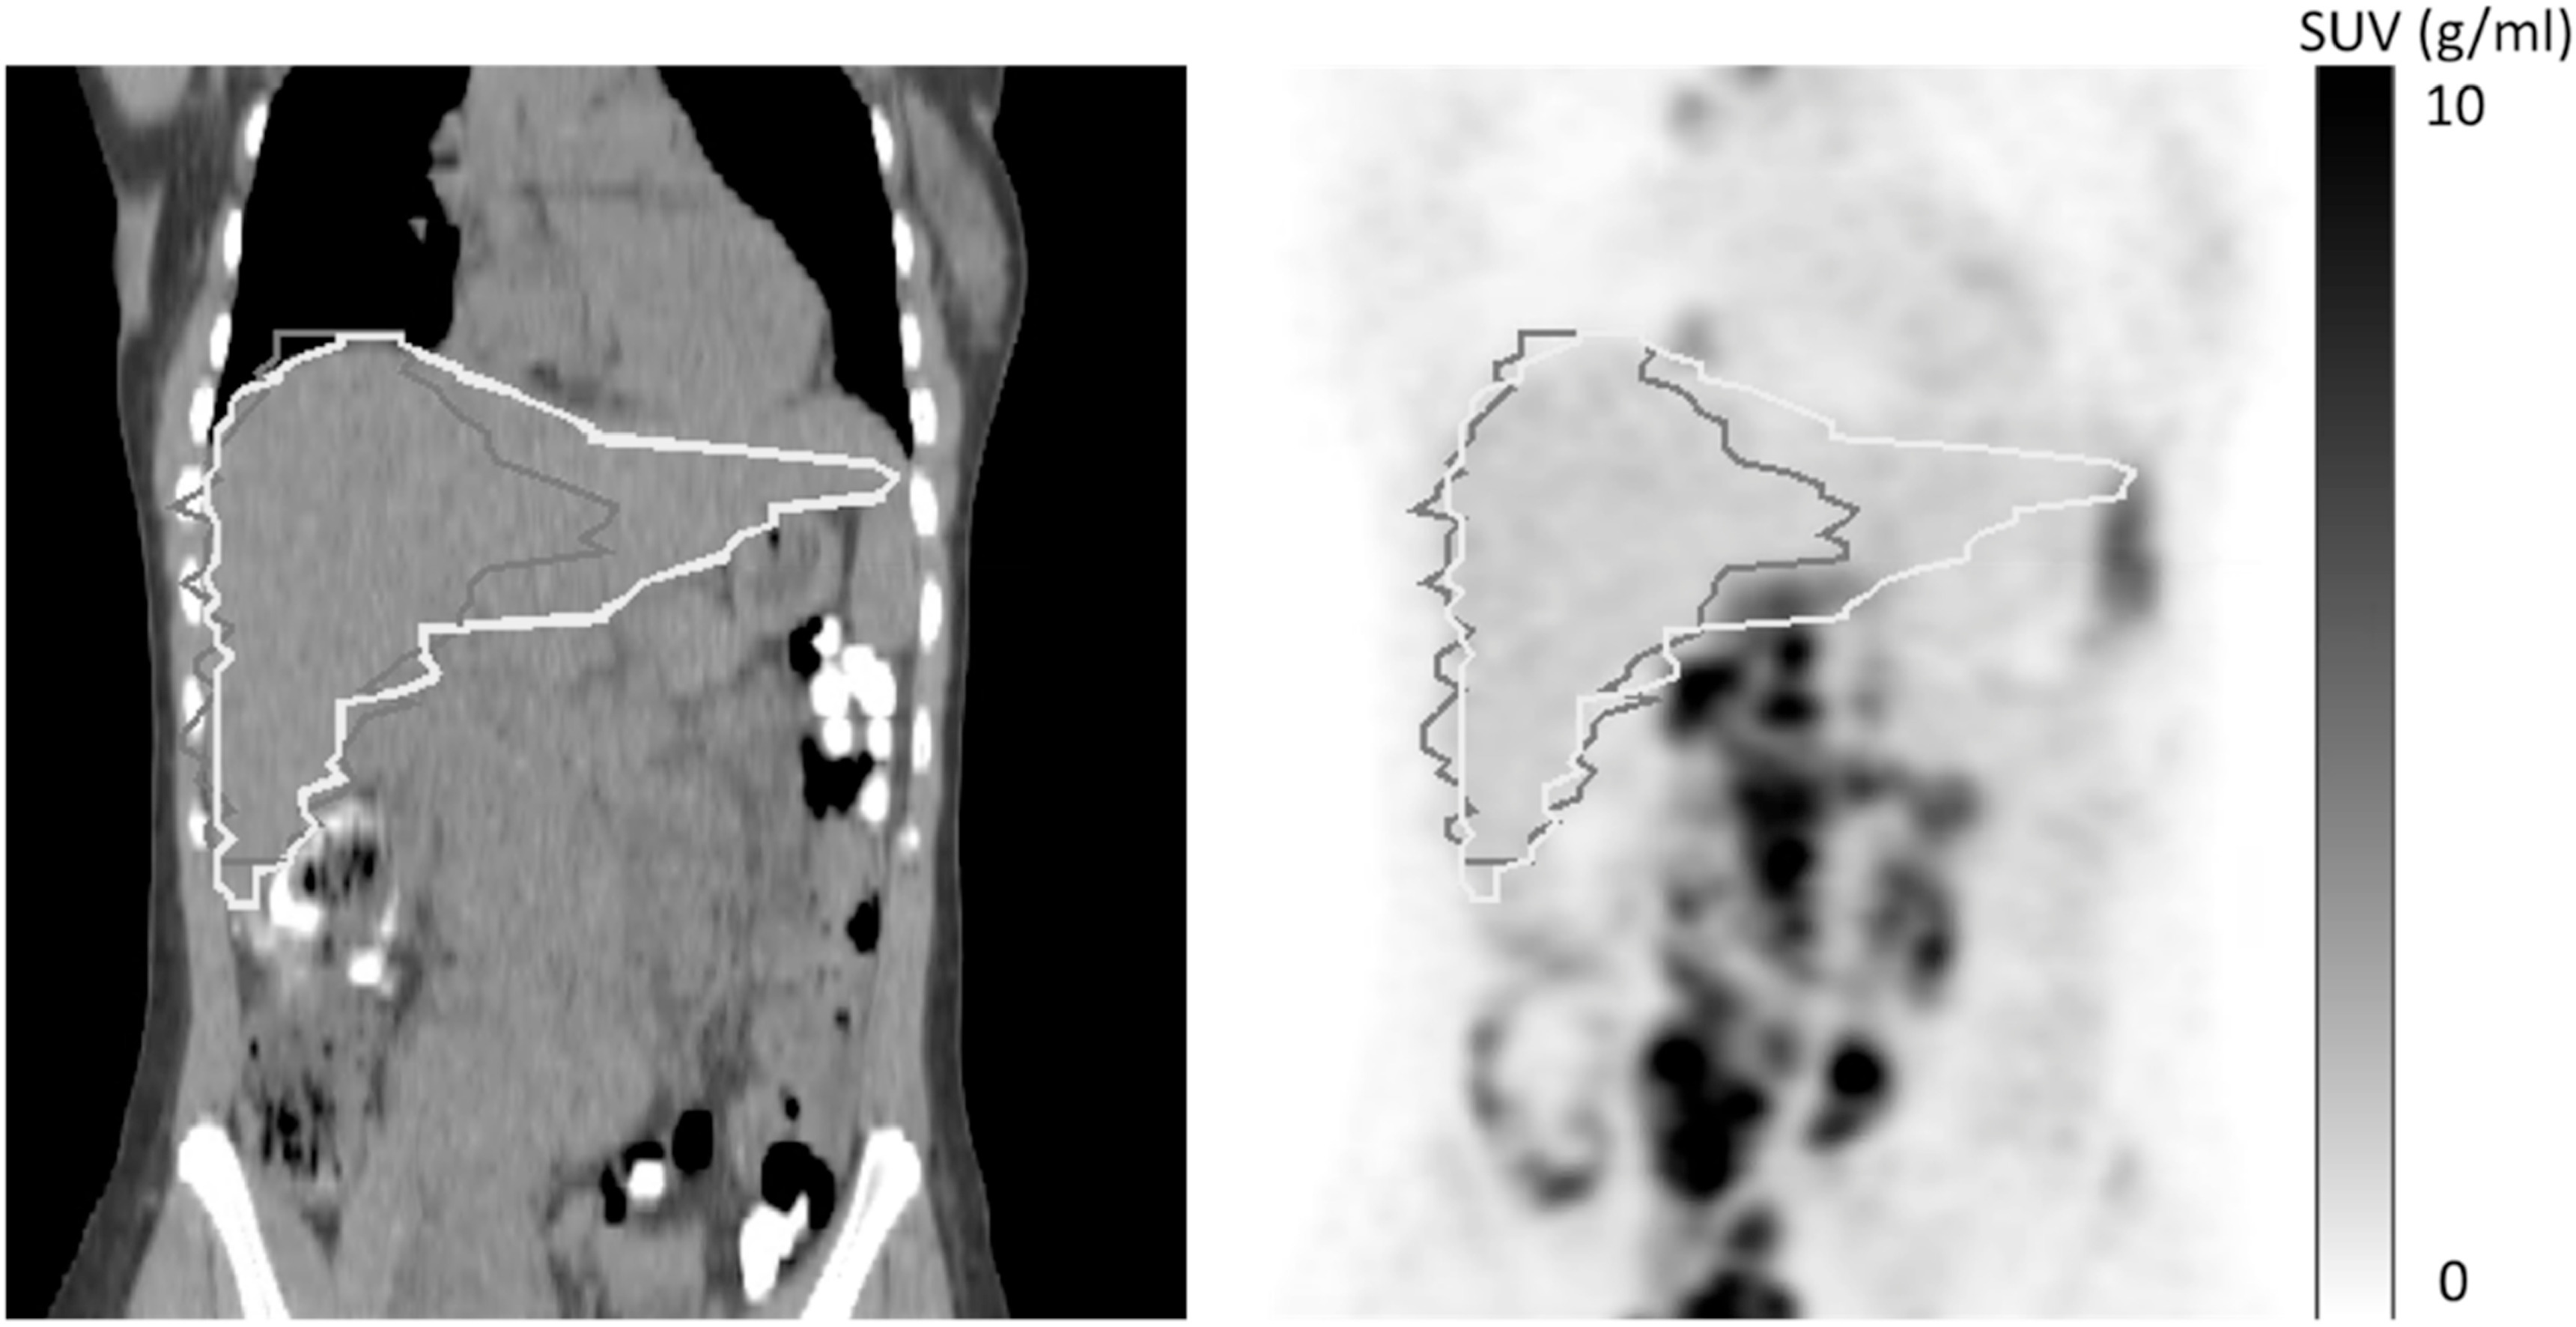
PET-man (brown) and CT-man (green) for the liver on a patient with poor agreement between the two techniques.
